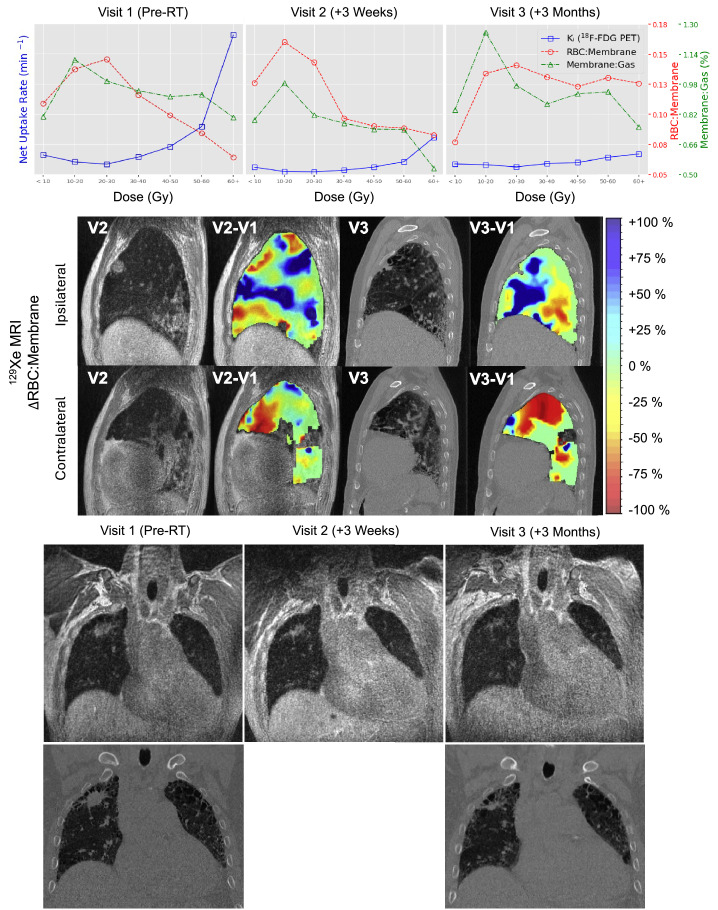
^18^F-FDG PET, HP-Xe MRI longitudinal and structural changes. ^18^F-FDG PET and HP-Xe MRI-derived metrics were compared (first row) before RT (left), and 3 weeks (middle) and 3 months after RT (right). Net influx constant, K_i_, describing the amount of irreversible ^18^F-FDG uptake is shown in blue, RBC:Membrane in red, and Membrane:Gas in green. Spatial maps depicting changes in RBC:Membrane with respect to the baseline visit (second row) are displayed with increases in RBC:Membrane shown in blue and decreases in red. UTE MR images (row 4) before RT (left), 3 weeks after RT (middle), and 3 months after RT (right) are shown. CT images (row 5; bottom) are shown for pre-RT (left) and 3 months post-RT (right). Changes in the tumor volume are depicted in both UTE and CT. Fibrotic changes near the tumor are observed 3 months post-RT. *Abbreviations:* 4D = 4-dimensional; CT = computed tomography; ^18^F-FDG = fluorodeoxyglucose; MRI = magnetic resonance imaging; PET = positron emission tomography; RT = radiation therapy; UTE = ultra short time to echo.
